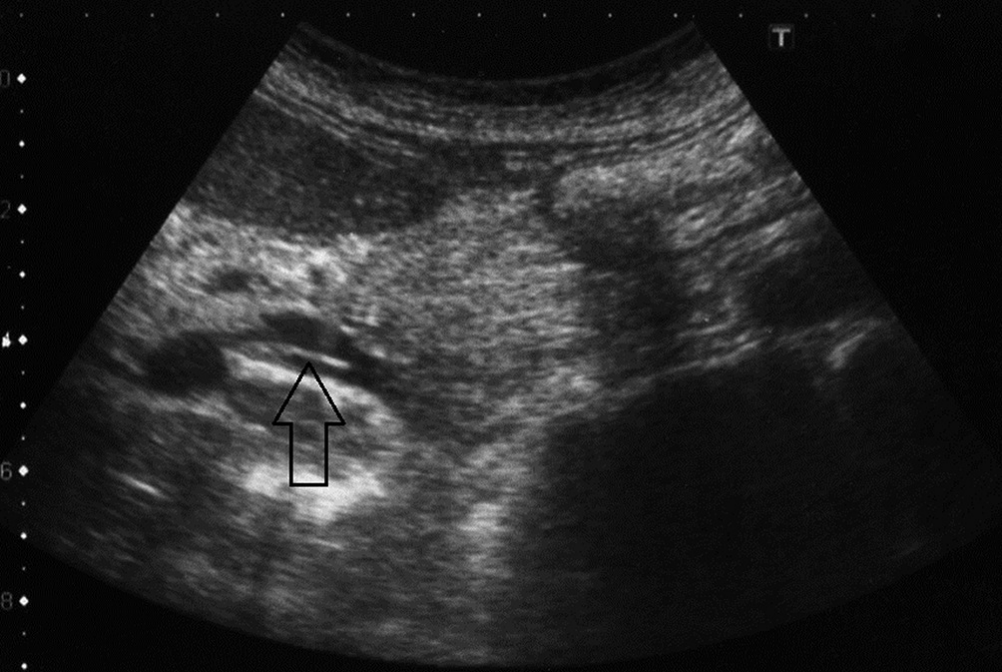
Ultrasound image reveals a thin, linear echogenicity, which is fixed at one end to the posterior wall of the common bile duct.
